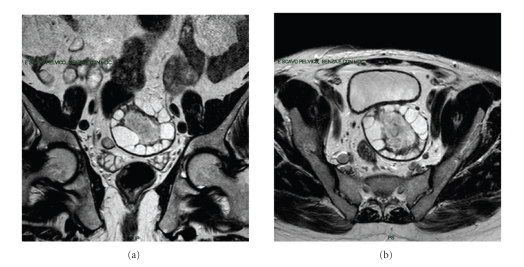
The MR images show the characteristic hydatid cyst in the retrovesical space, adherent to the sigmoid colon.
